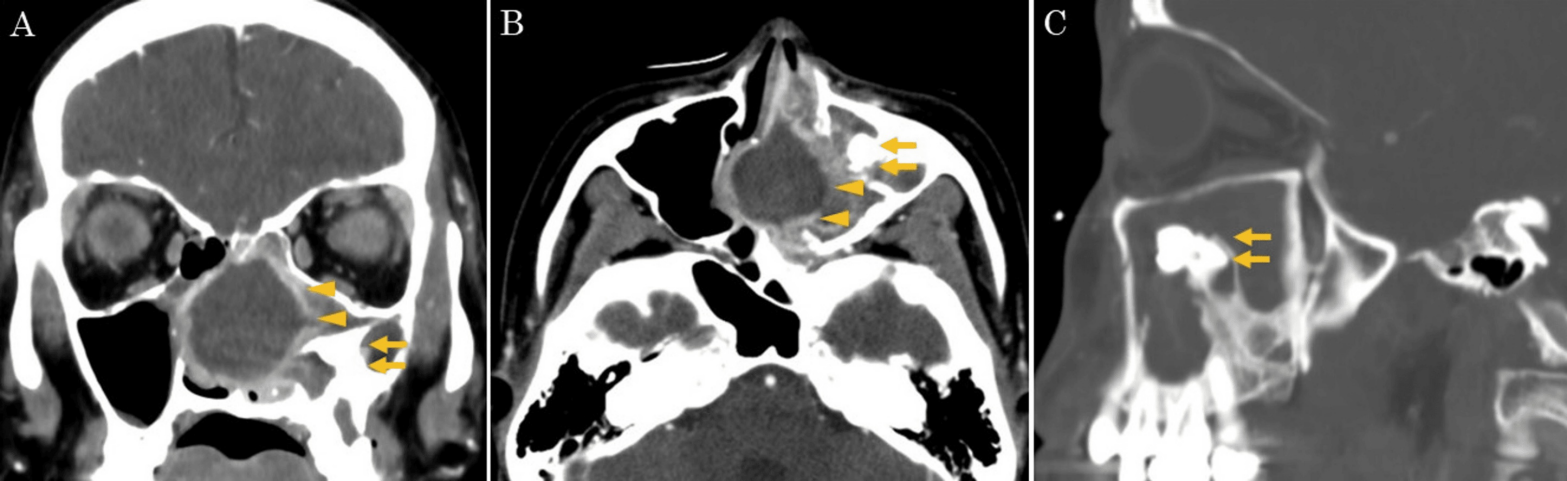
Contrast-enhanced sinus CT images at the first visit Coronal (A) and axial (B) images show a mass lesion with enhanced margins in the left nasal cavity and maxillary sinus (arrowhead) and an ectopic tooth adjacent to the lesion (arrow). The perpendicular plate of the ethmoid bone is destroyed. Sagittal CT in the bone window image (C) show the ectopic tooth eruption in the floor of the maxillary sinus. CT: computed tomography
